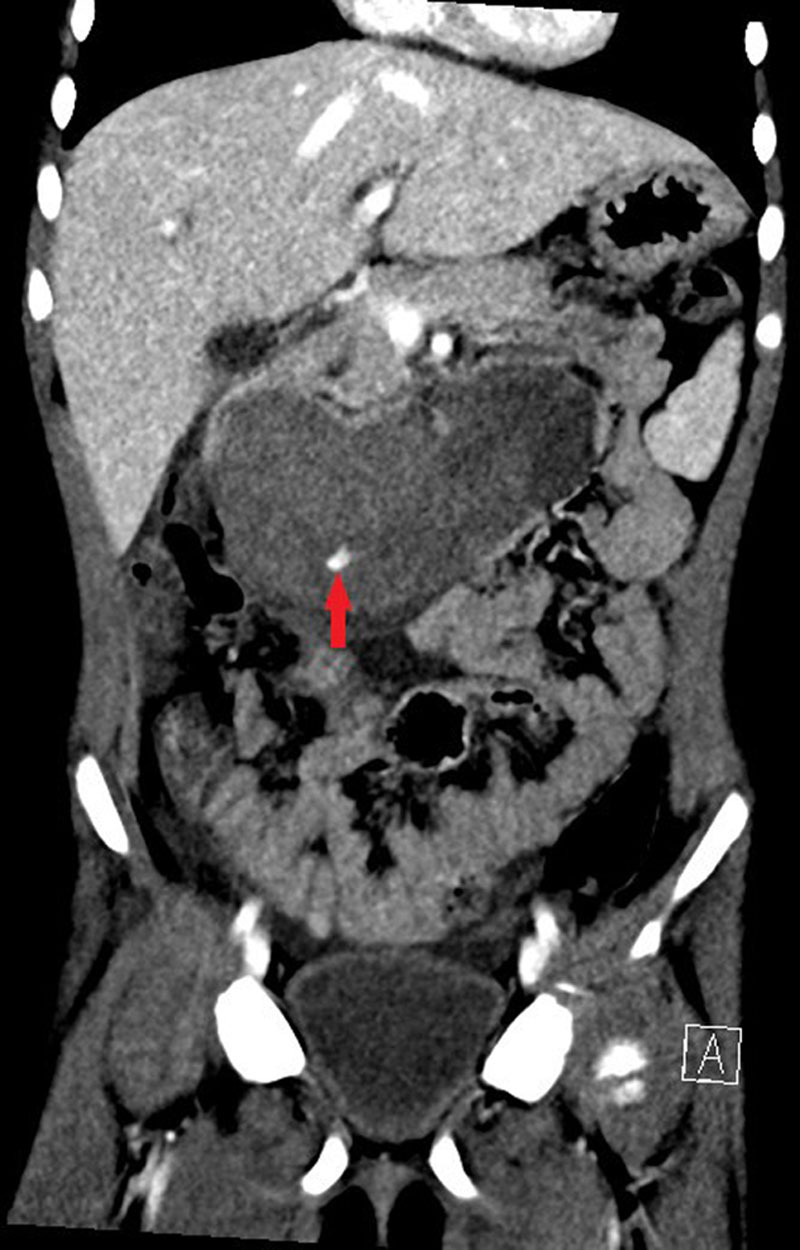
Coronal CT image of abdomen demonstrates a large intramural hematoma in second and third part of duodenum with focal contrast extravasation (arrow) consistent with active bleeding.
